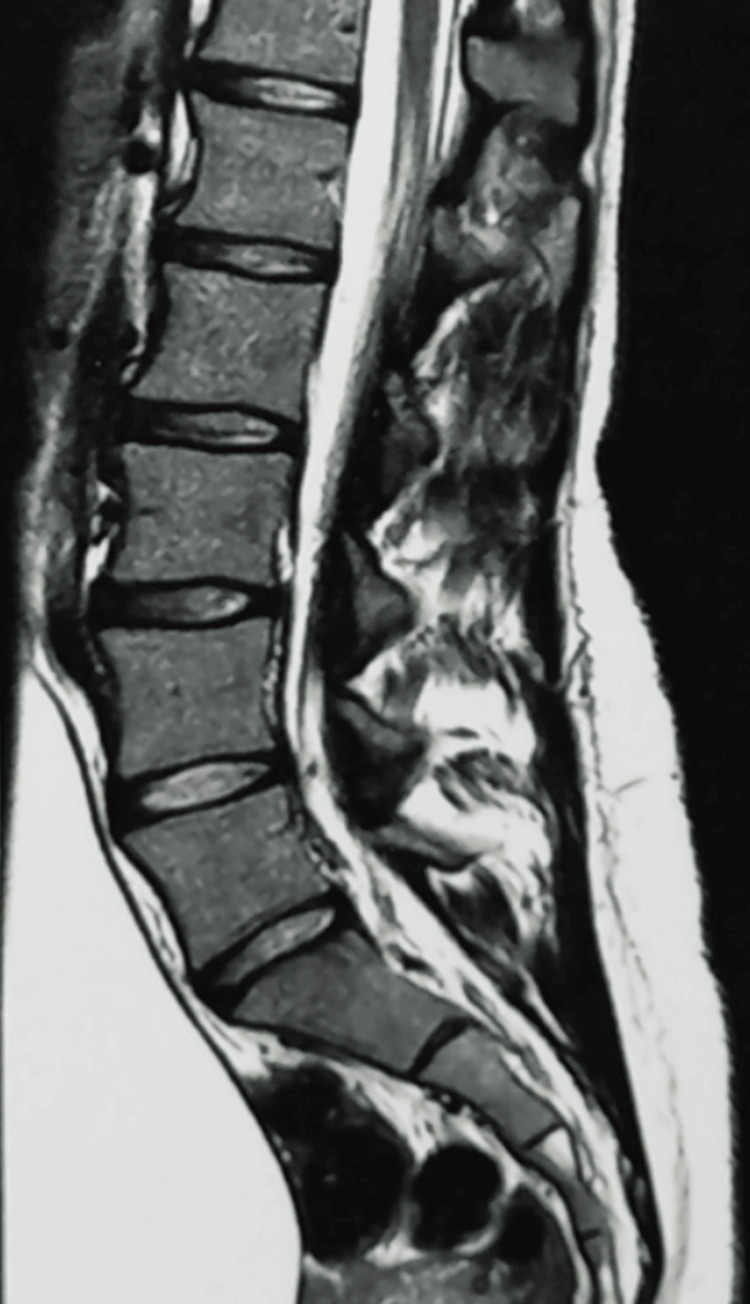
Neuroaxis MRI in sagittal section In this image of the lumbosacral region, we can observe the mass located anterior to the vertebrae. MRI: magnetic resonance imaging
